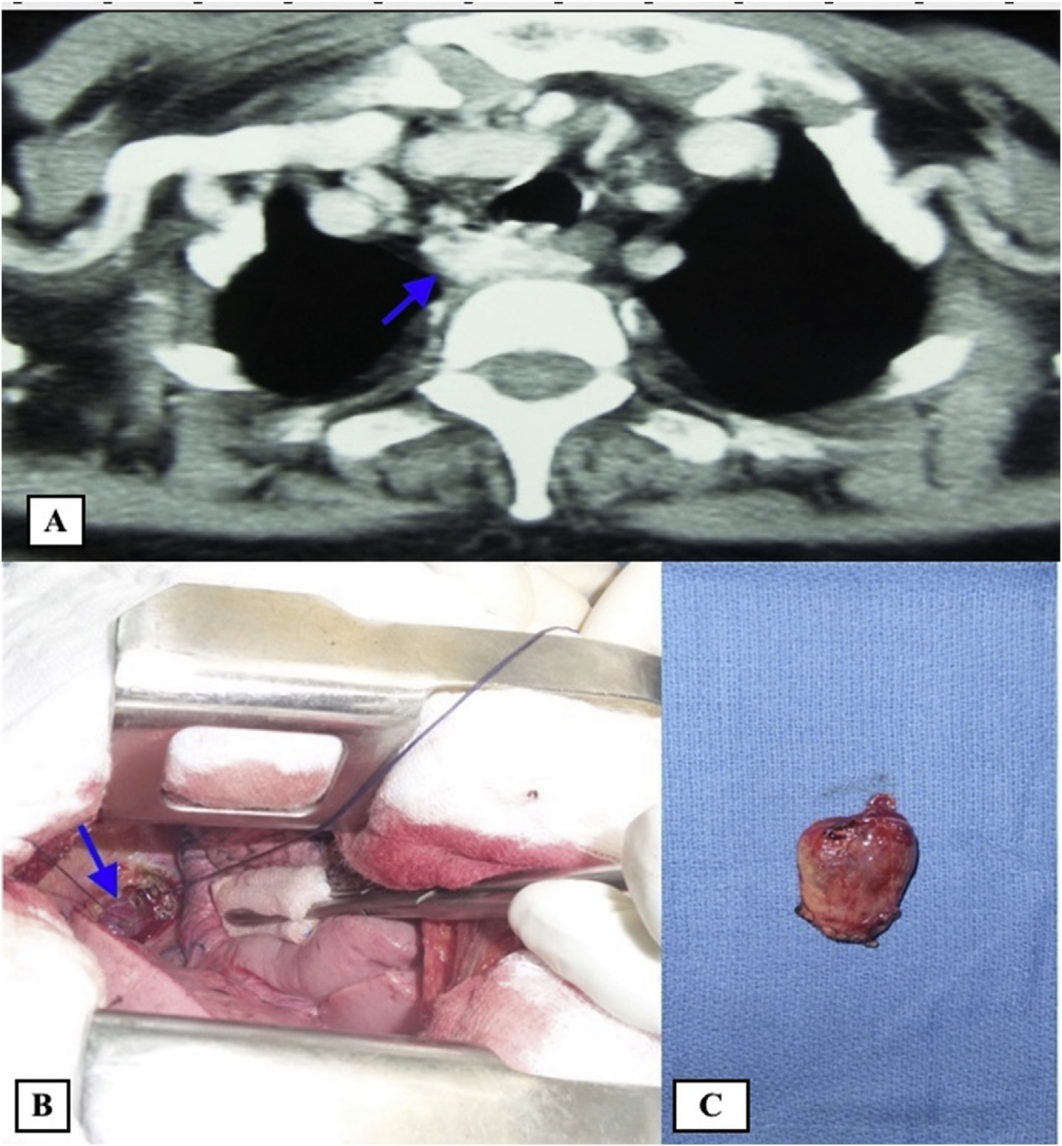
A: Thoracic CT-scan showing a retrotracheal mass with enhancement after contrast injection. B: Intraoperative view of the tissular mass described above. C: Macroscopic view of the lesion after surgical excision.
